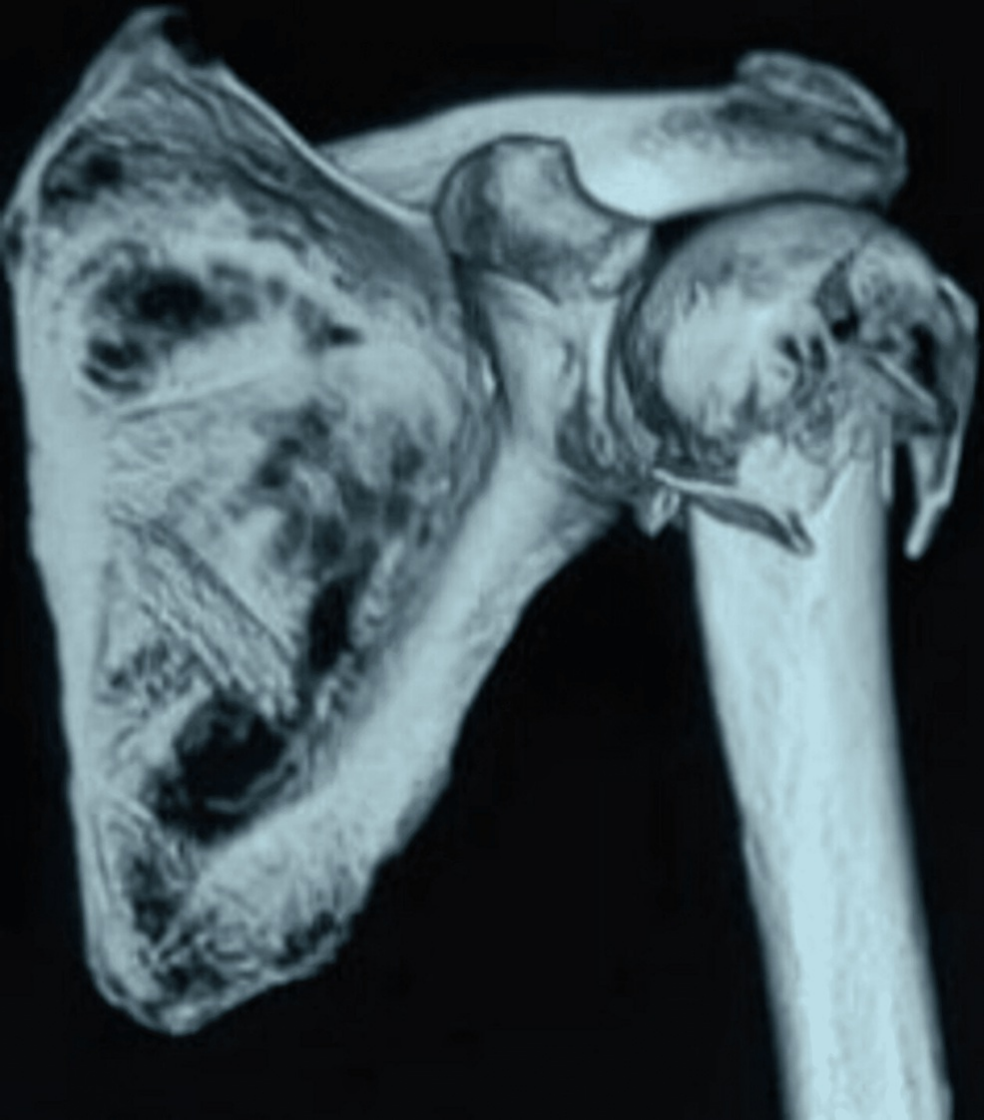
CT scan showing three-part communited proximal humerus fracture
